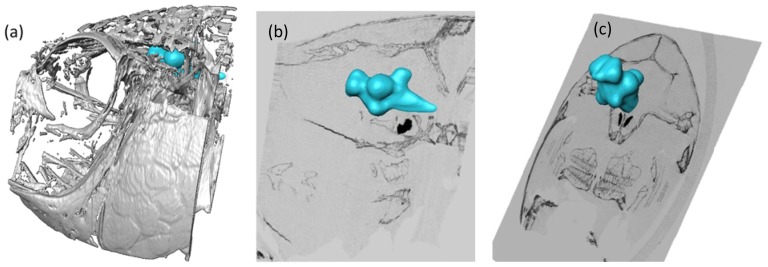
Overlap of MRI brain images (blue) with CT head data (light grey) in the Amira environment. (a) depicts a 3D reconstruction of the tilapia head based on the CT data set overlaid with a 3D tilapia brain. (b) and (c) show 2D sections of the head CT (sagittal and transverse views, respectively) and the tilapia's brain position in those perspectives.
